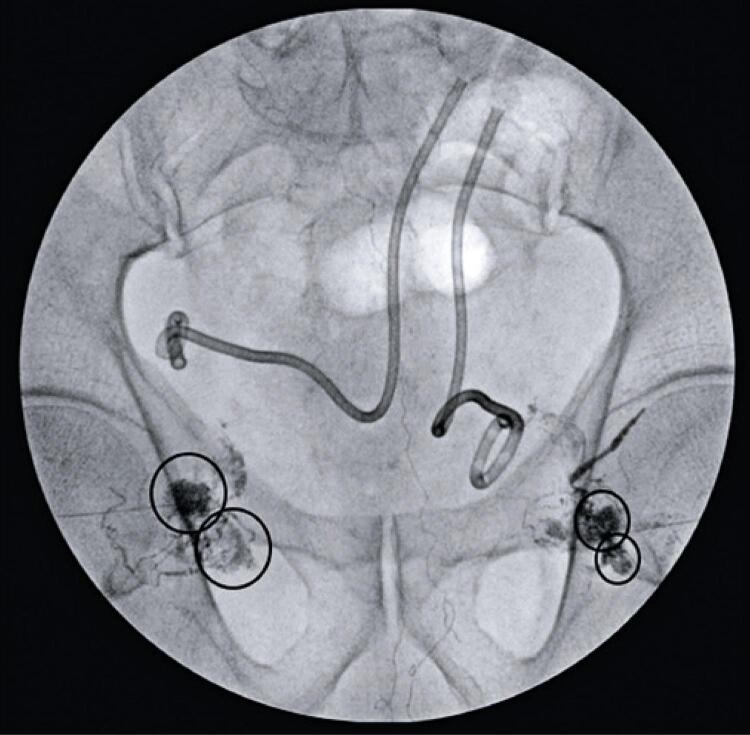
Pelvic radioscopy showing lymph nodes with enhancement (black circles) after ultrasound-guided puncture and lipiodol infusion
